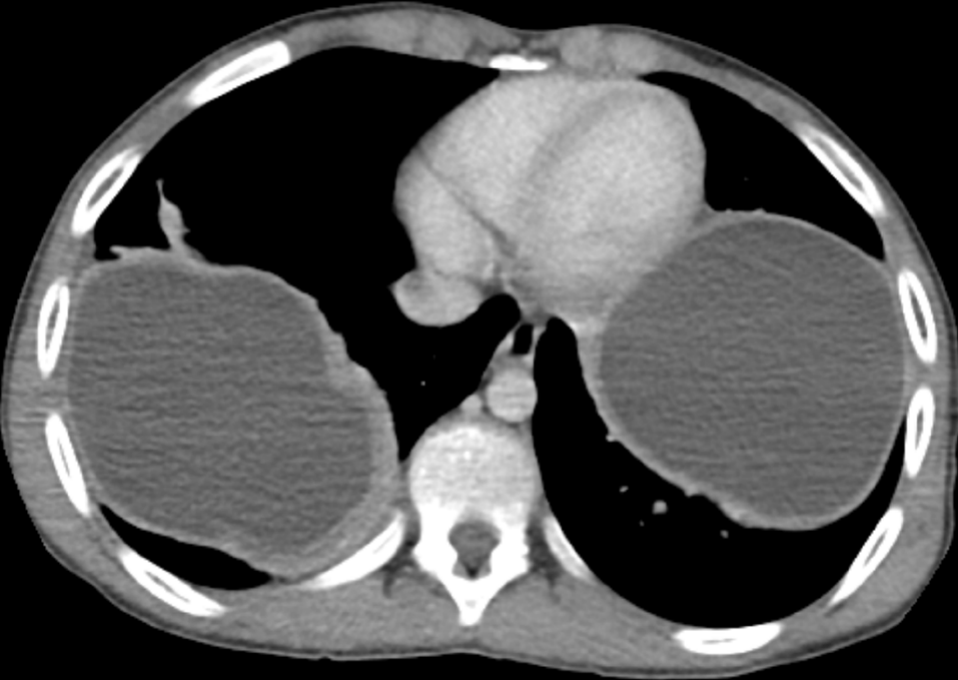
In a 13-year-old male, the image of giant hydatid cysts located in the lower lobe of the right lung and the lower lobe of the left lung is seen in the thorax computed tomography mediastinal window.
